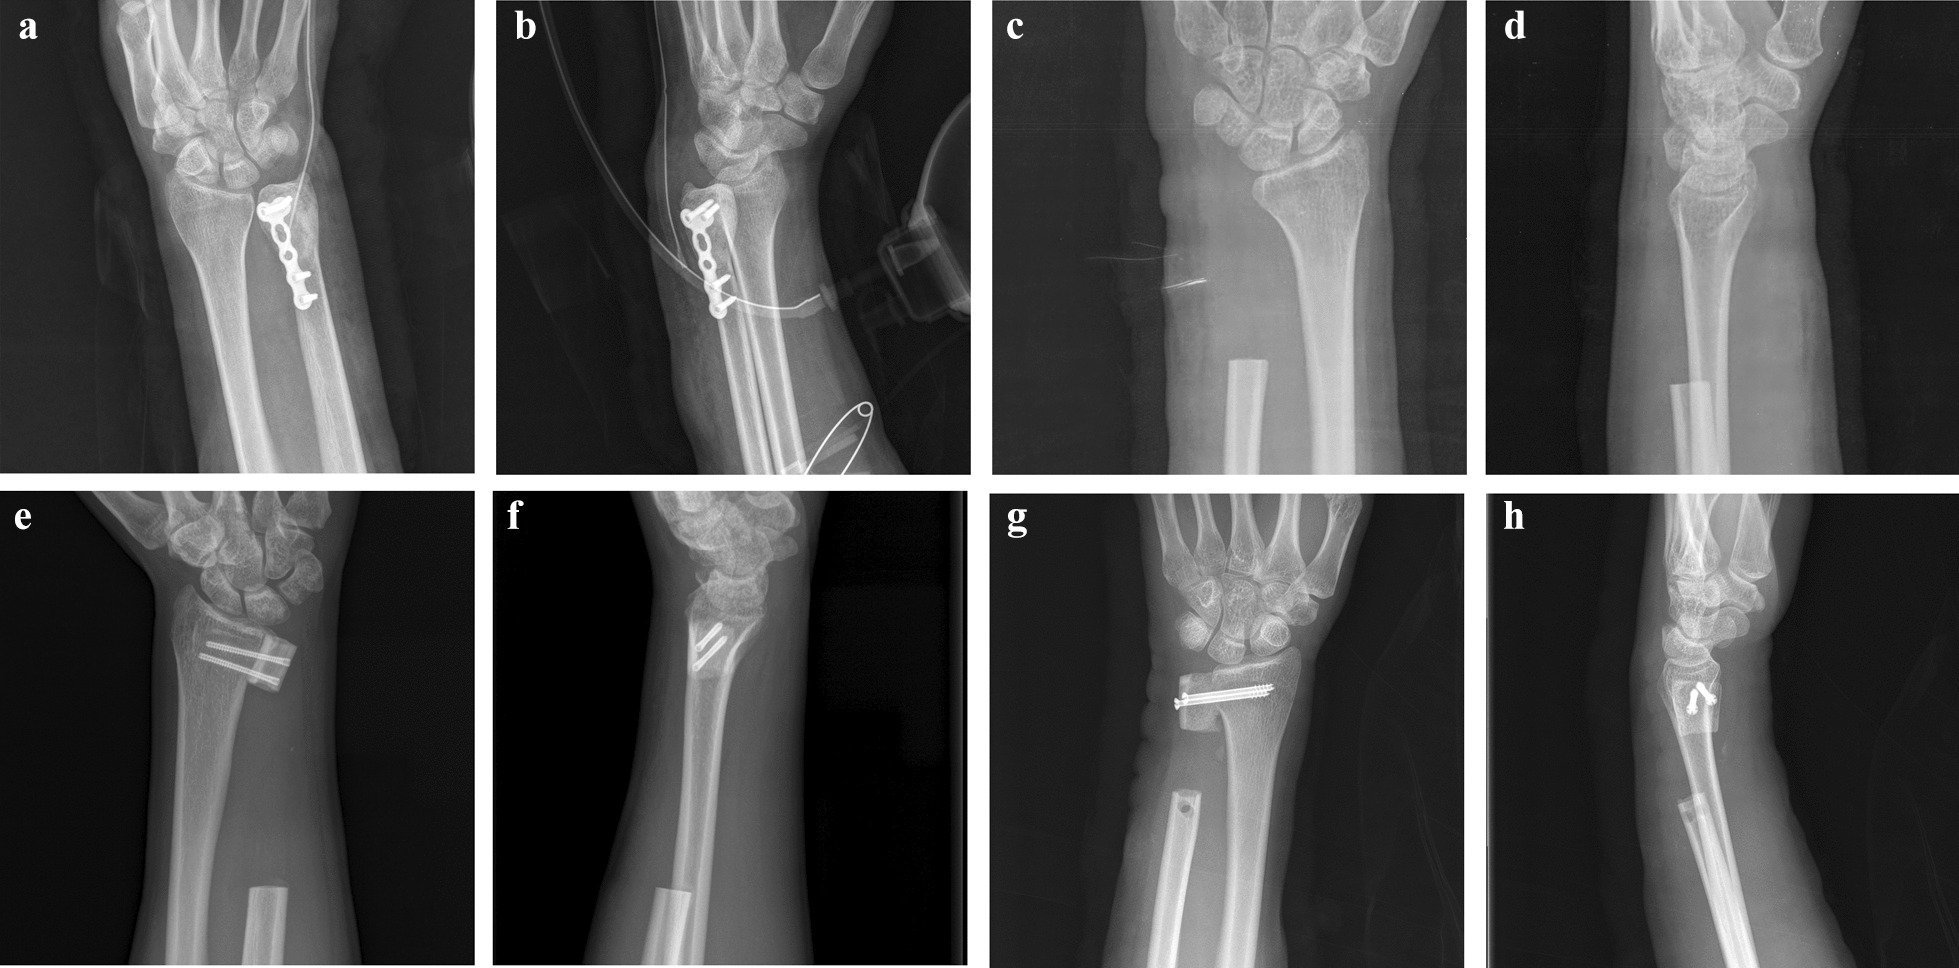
A, B Postoperative X-ray images of extended intralesional curettage. C, D Postoperative X-ray images of the Darrach procedure. E, F Postoperative X-ray images of the original Sauvé–Kapandji procedure. G, H Postoperative X-ray images of the modified Sauvé–Kapandji procedure with ECU tenodesis
